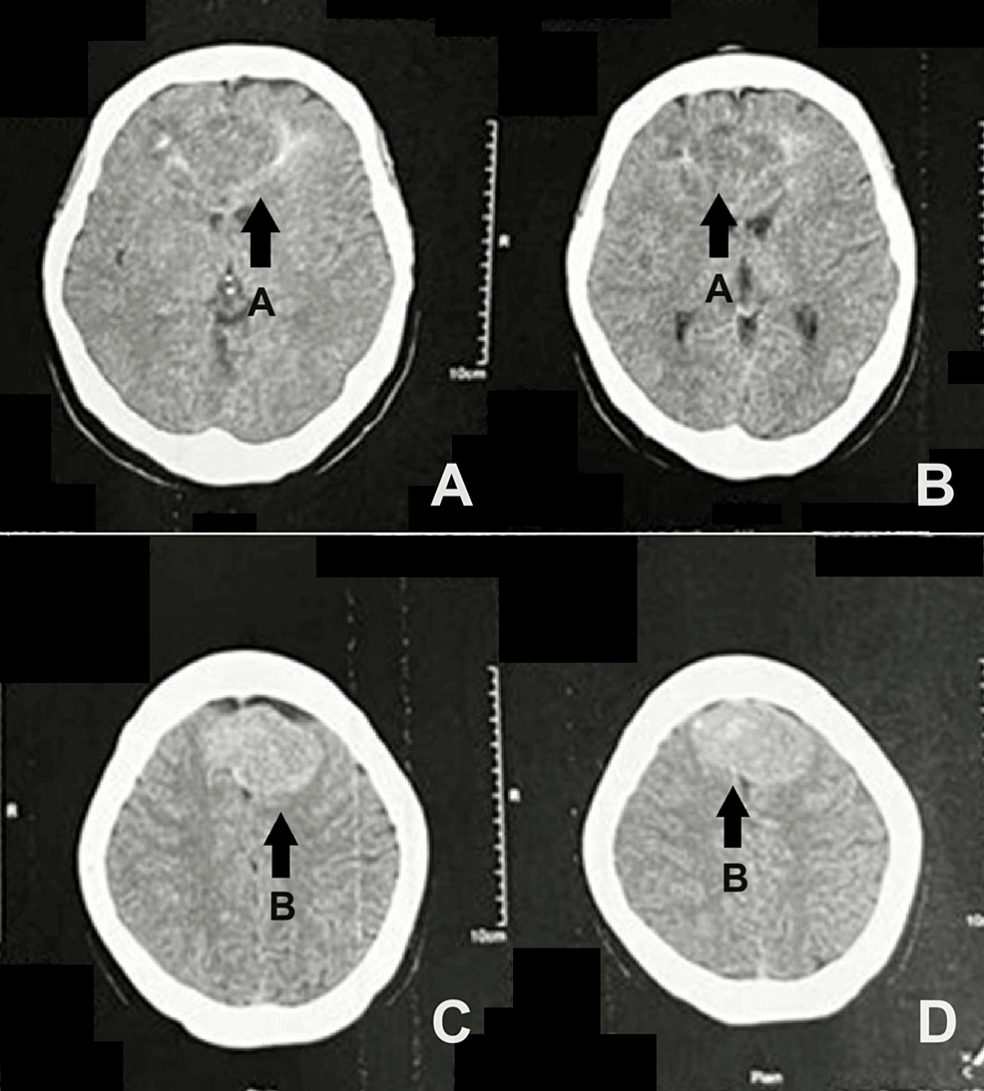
Axial cranial CT scan without contrast of the patient. Arrow A in panels A and B points to a fairly defined, heterogeneous, intra-axial mass measuring 3.1x4.2x3.4 cm, crossing the midline in both frontal lobes with perilesional edema. Arrow B in panels C and D points to a well-defined, iso- to slightly hyperdense, extra-axial mass adjacent to the falx cerebri measuring 3.4x4.8x3.4 cm, with minimal calcifications in the mid-frontal convexity with minimal perilesional edema.
